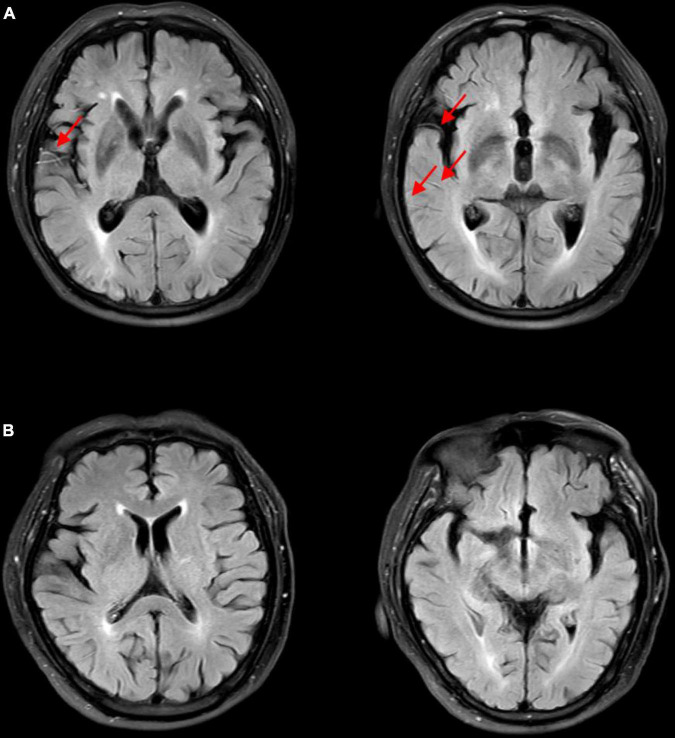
FLAIR images of representative “high” and “low” FVH scores in acute stroke patients. (A) High FVH scores (FVH = 6). Red arrows show FVHs. (B) Low FVH scores (FVH = 0). FVH, fluid-attenuated inversion recovery vascular hyperintensity.
